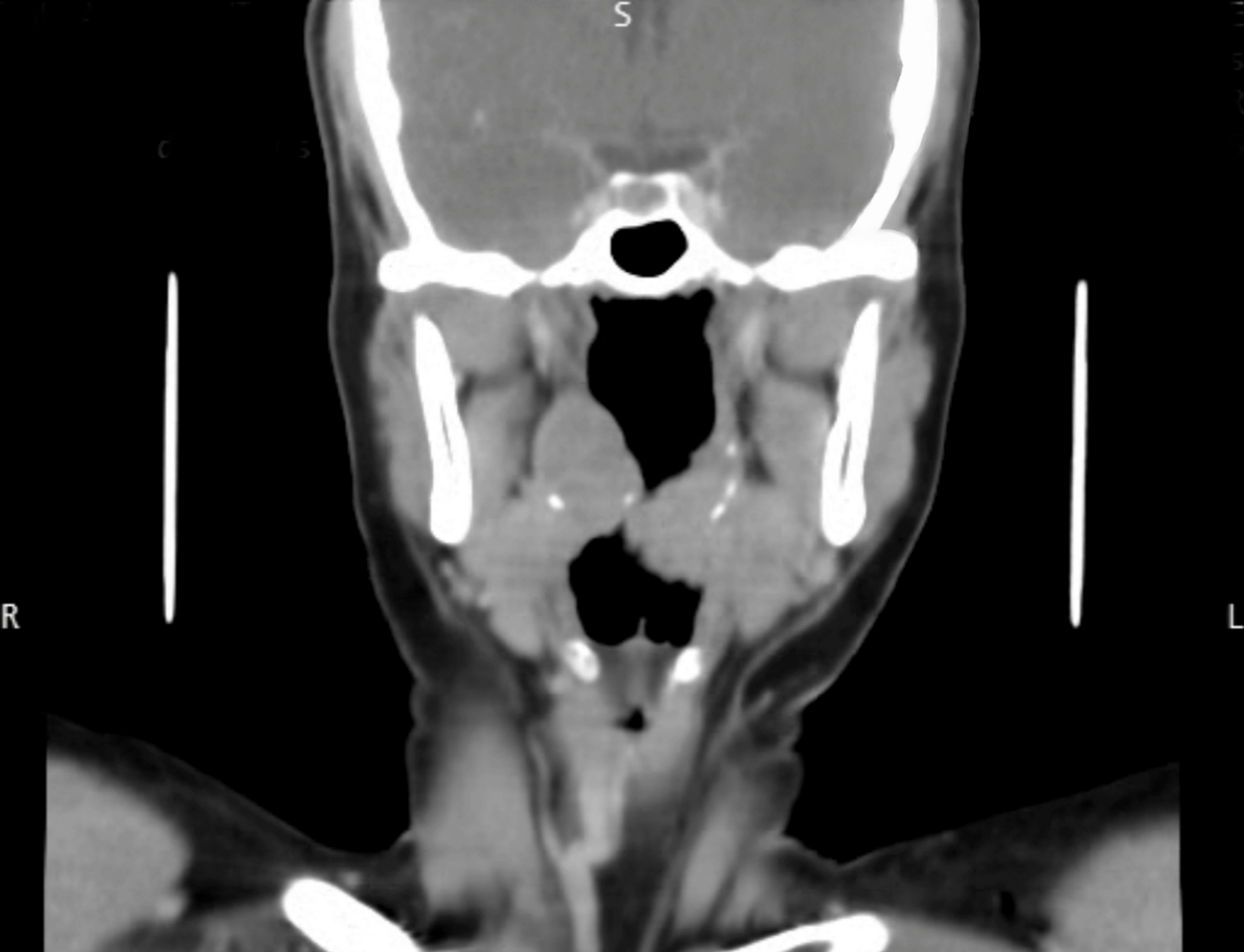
Coronal section of contrast-enhanced CT scan showing bilaterally enlarged tonsils with heterogeneous post-contrast enhancement and foci of peripheral calcification
